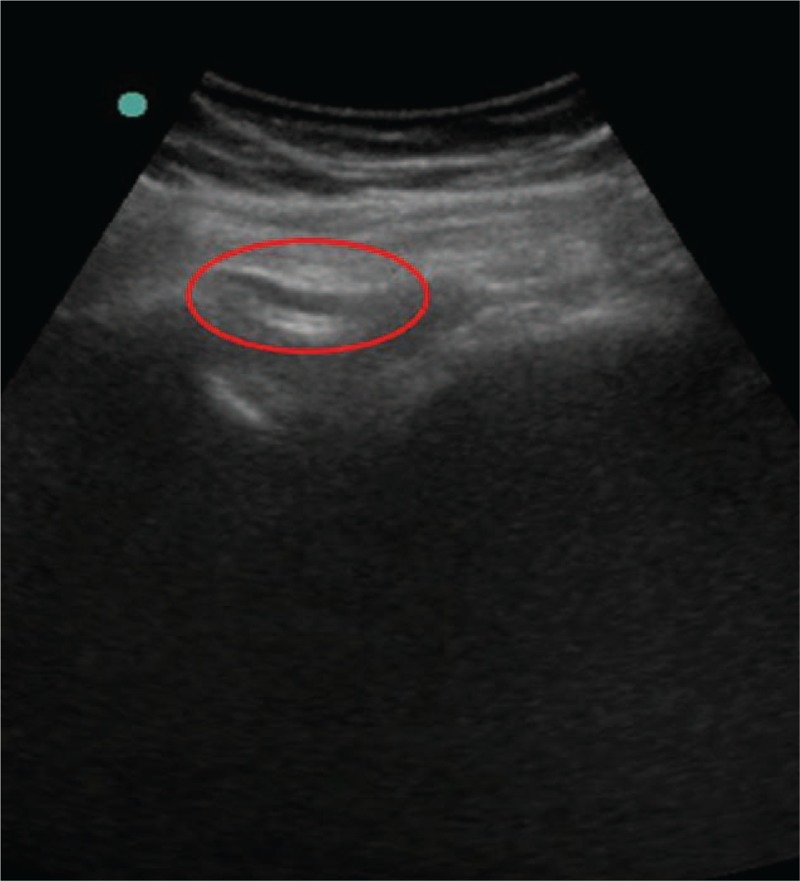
Ultrasound image of the sciatic nerve. The local anesthetic is deposited under the gluteus maximus muscle, and the sciatic nerve is highlighted (circle).
